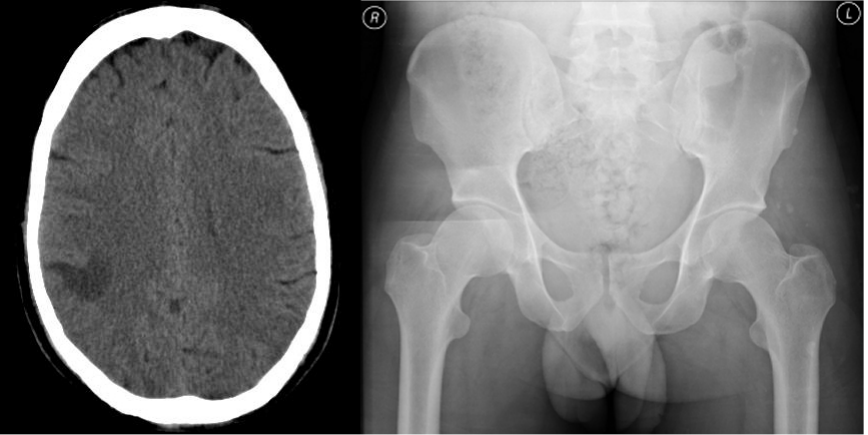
Head CT scan at presentation and pelvic plain film x-ray. Left: Head CT showing an area of subcortical reduced attenuation that could be mistaken for ischaemia. Right: Pelvic x-ray demonstrating several small, discrete, calcified lesions in the left gluteal muscles.
